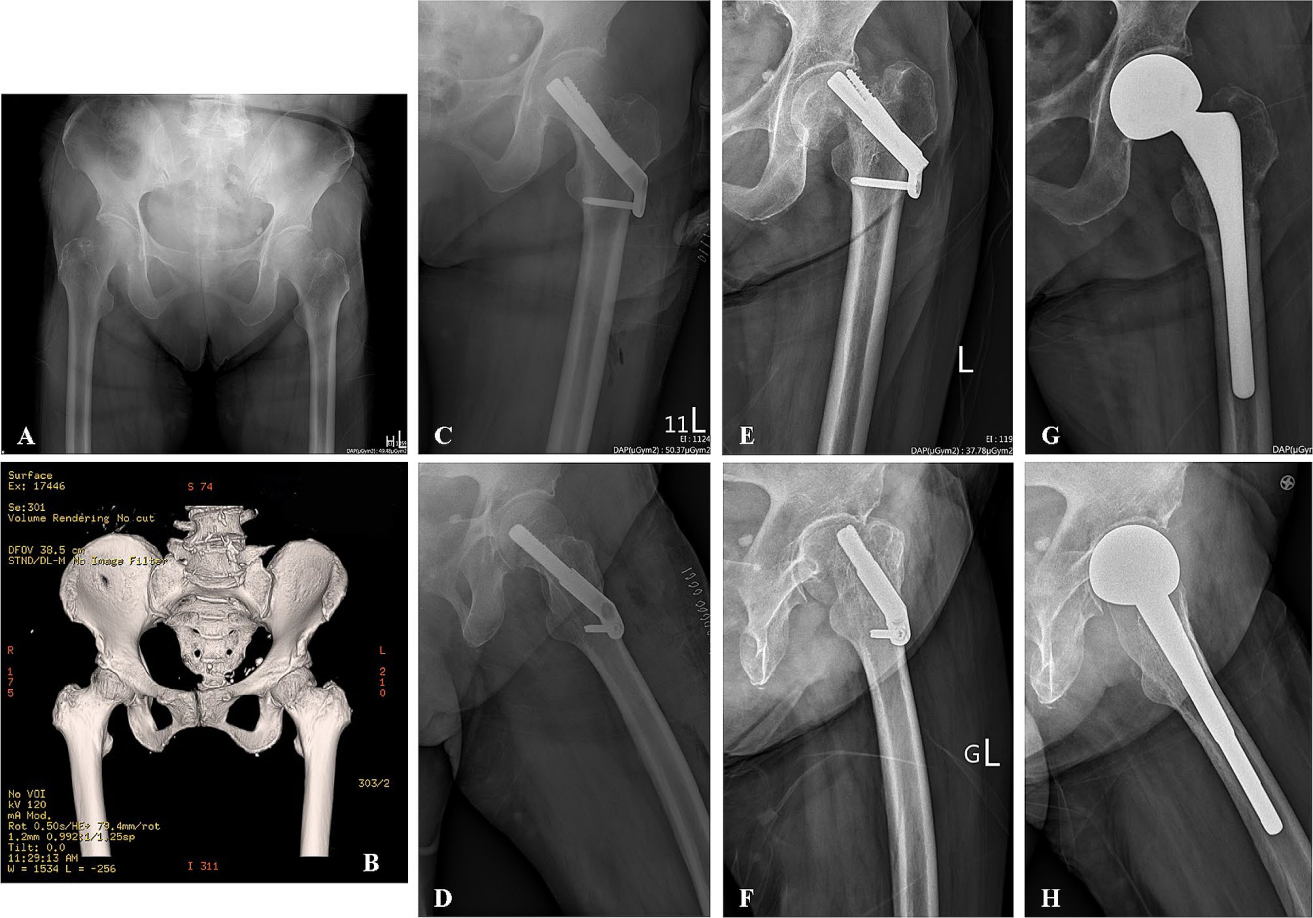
A 74 y/o woman with subcapital femoral neck fracture (Pauwel Type II): A Preoperative AP view; B Preoperative 3D reconstruction CT scan; C Immediate postoperative AP view; D Immediate postoperative lateral view; E Postoperative AP view at 8 months; F Postoperative lateral view at 8 months; G Post-hemiarthroplasty AP view; H Post-hemiarthroplasty Lateral view
